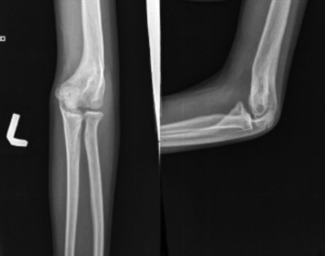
Post-operative antero-posterior and lateral radiograph of the case above in Figure 1 after 12 months of operation.
